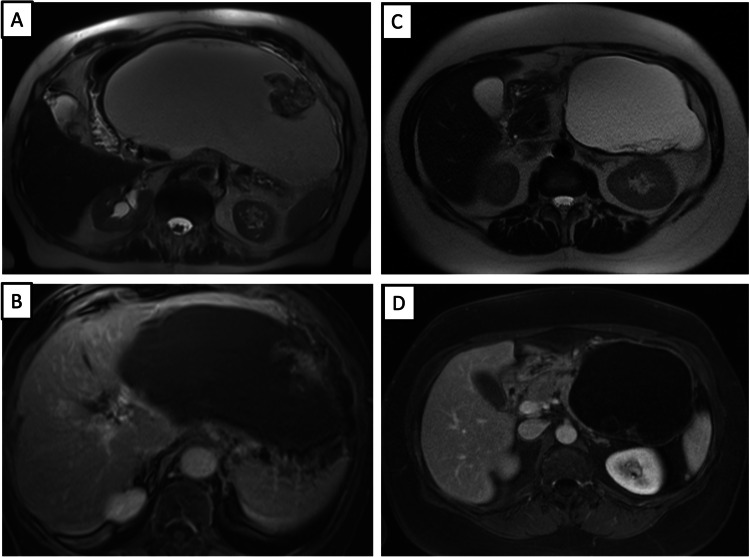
Comparison of lymphoepithelial cyst (LEC) and mucinous cystic neoplasm (MCN). (a, b) A 47-year-old female patient with huge LEC in the pancreatic body and tail, lesion size 22 cm. Axial T2-weighted MR image without fat suppression (a) and T1-weighed MR image in the venous phase (b) show a LEC with mural nodules and enhancing solid component in the ventral wall of the lesion (size of the solid component 5,6 cm). (c, d) An identical image to a mucinous cystic neoplasm in a different patient. Axial T2 weighted MR image without fat suppression (c) shows a big MCN in the pancreatic body and tail with septations in the dorsal parts. Axial T1-weighted MR image with fat suppression (d) shows an enhancing solid component in the dorsal parts of the cystic lesion
